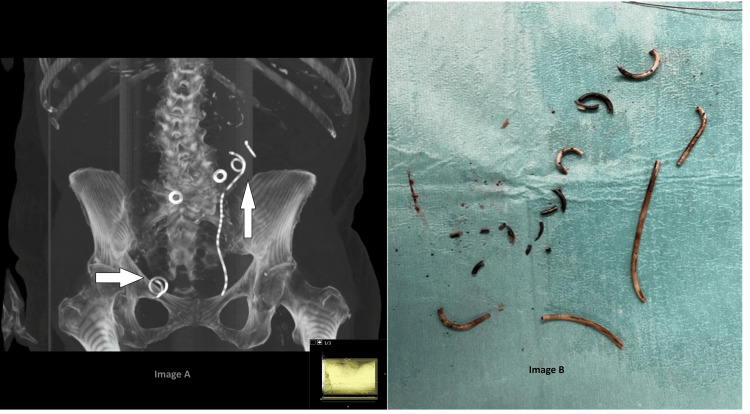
Image A shows a MIP (maximum intensity projection image) from a coronal section of a CT abdomen and pelvis showing a modified bone window with pieces of fragmented stent coils in the kidney (vertical arrow) and bladder (horizontal arrow), and Image B shows the stent removed in numerous pieces. (A) Maximum intensity projection MIP CT image of the abdomen and pelvis with a modified bone window showing multiple fragmented coils of a double J ureteral stent in the kidney (vertical arrow) and bladder (horizontal arrow).
